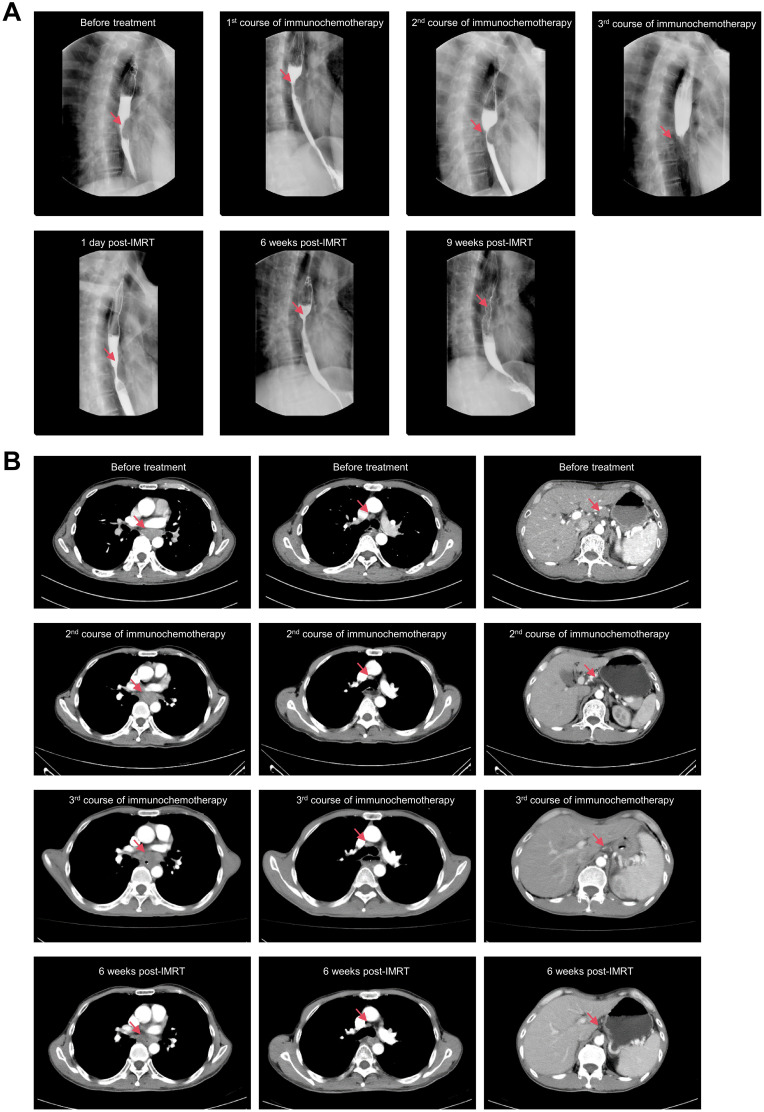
Radiographic evaluations during treatment. (A). X-rays with barium contrast of esophagus. (B). Chest CT scan with contrast.
